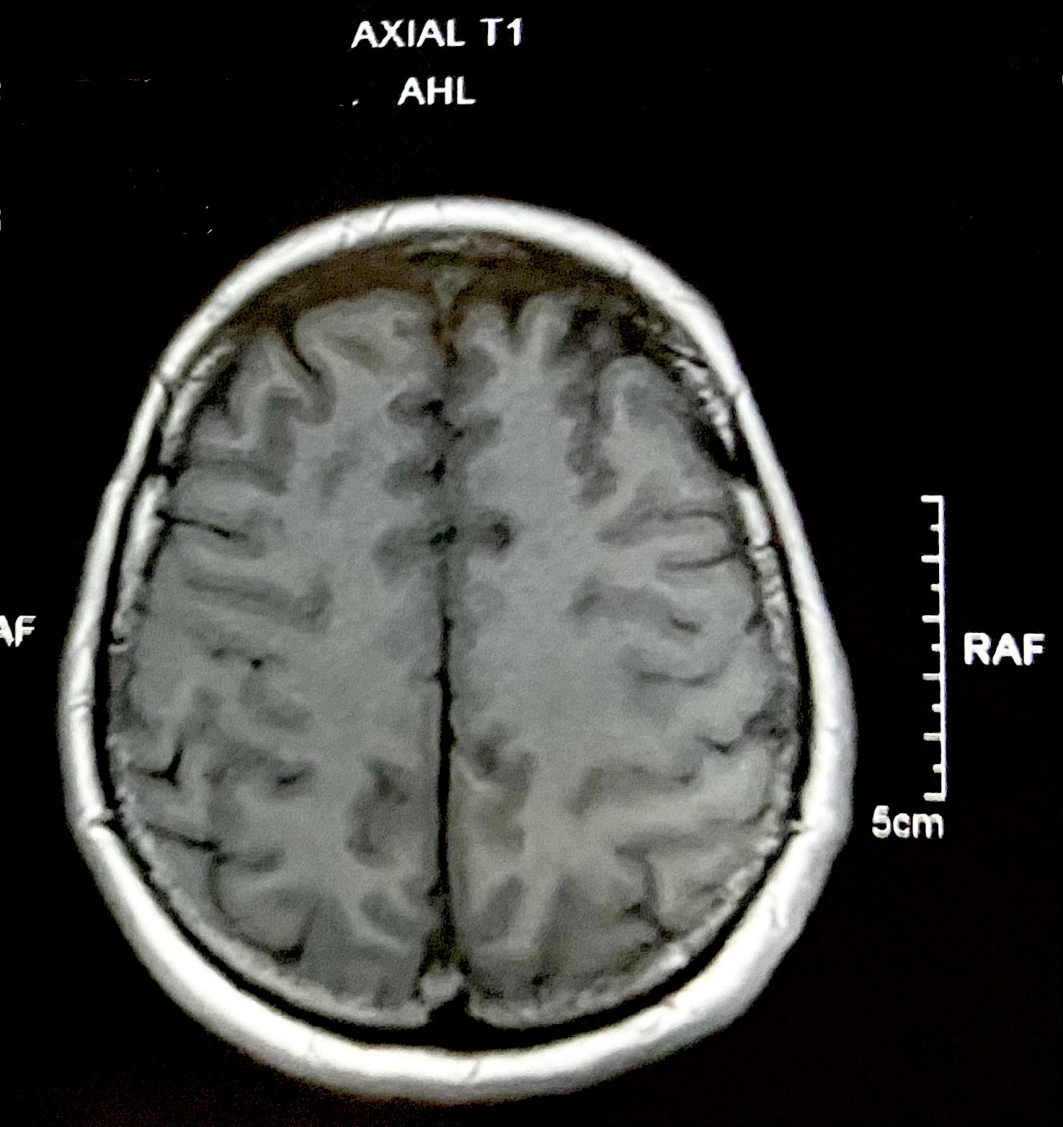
Axial T2 view of MRI brain.
